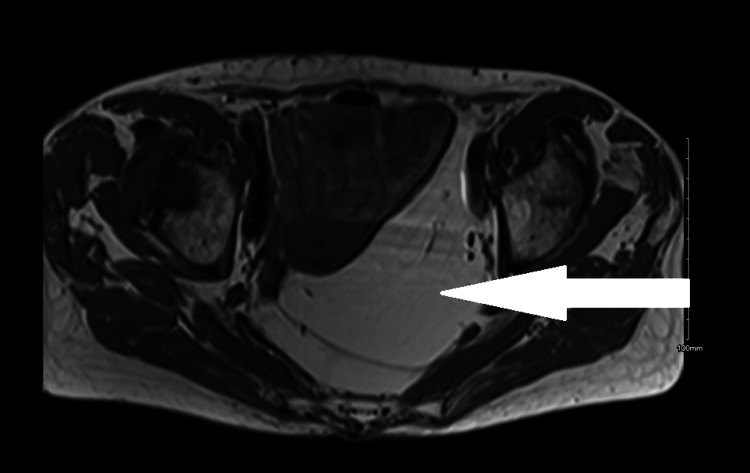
Axial view of pelvic lipoma on magnetic resonance imaging.
